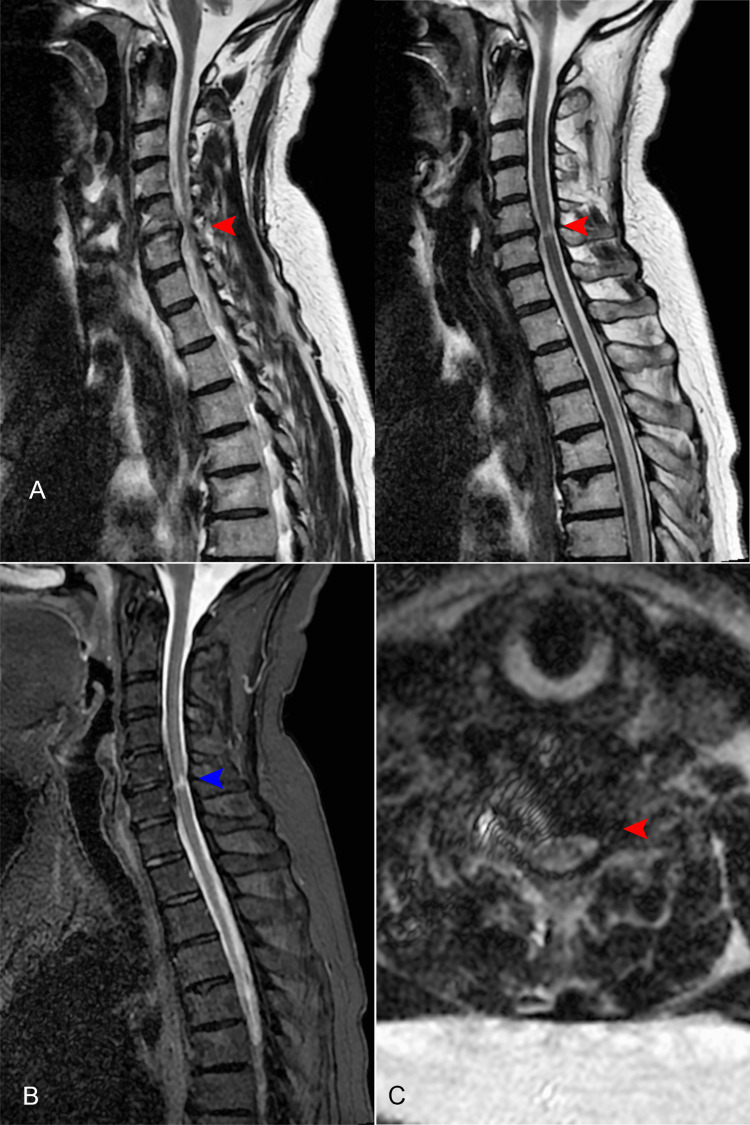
Preoperative MRI showing cervical disc herniation at the C5-C6 level compressing the spinal cord (red arrows) and signs of cervical myelopathy (blue arrow) due to disc compression on the spinal cord. (A) T2 sagittal section; (B) short tau inversion recovery sagittal section; (C) T2 axial section.
